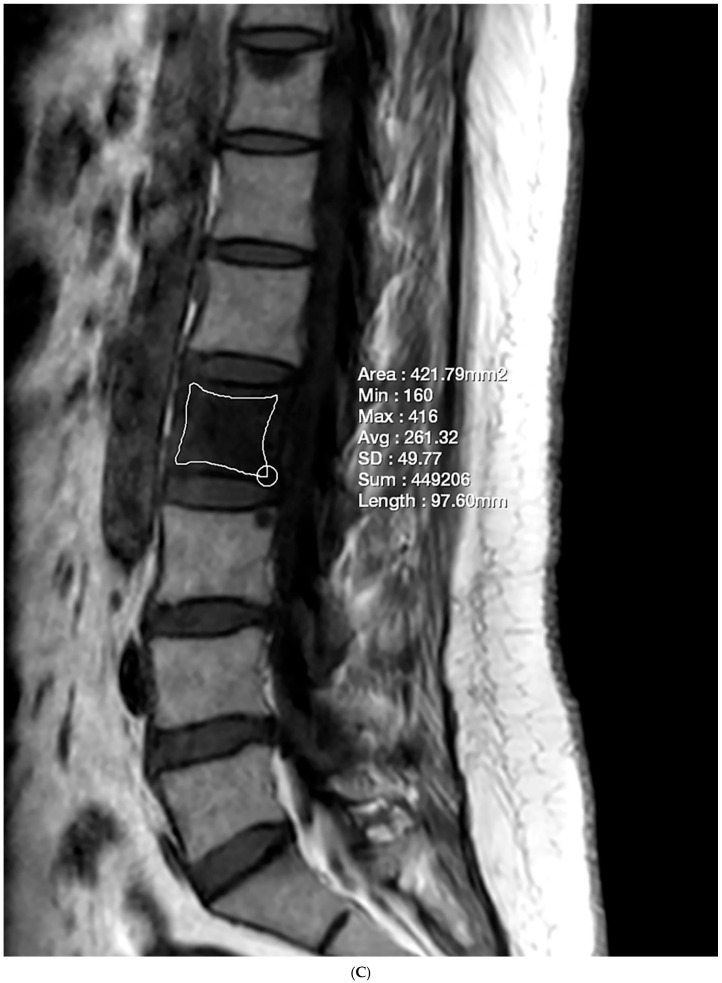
Lumbar spine MRI of a 48-year-old female with multiple bone metastases from adenoid cystic carcinoma of the parotid gland. (A) GRE-based CSE-MRI reveals an FF of 1.05% in the metastatic lesion of the L2 vertebral body. (B) On SE-based CSE-MRI, the FF of the corresponding lesion was 7.30%. The number in the figure indicate the ROI numbering in the EXPRESS program. (C) Multiple bone metastases are observed in the T11, L2, and L3 vertebral bodies on the T1-weighted image. The VBQ score for the L2 vertebral body lesion was 0.96.
